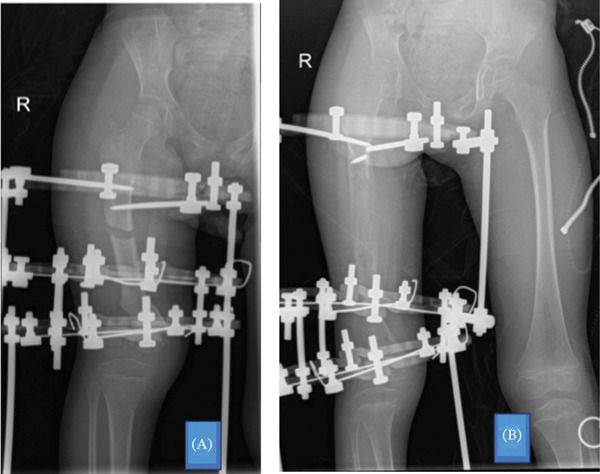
Serial AP radiographs during distraction at (A) 2 weeks and (B) 12 weeks showing gradual opening of the distal wedge and progressive lengthening at the midshaft level with visible regenerated bone formation.
